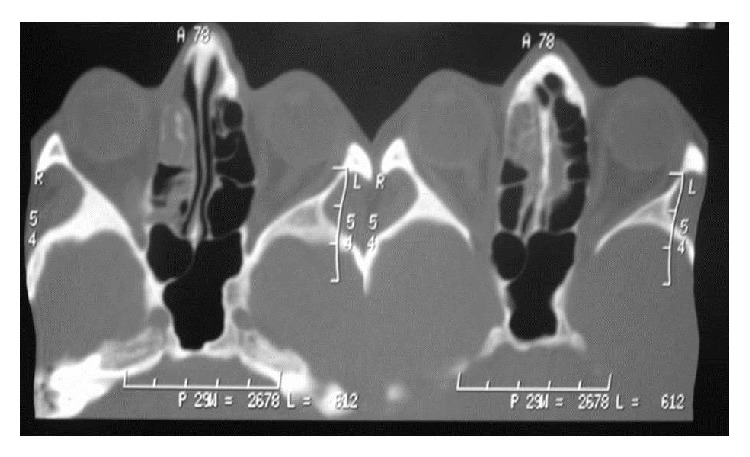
CT section of the region.
